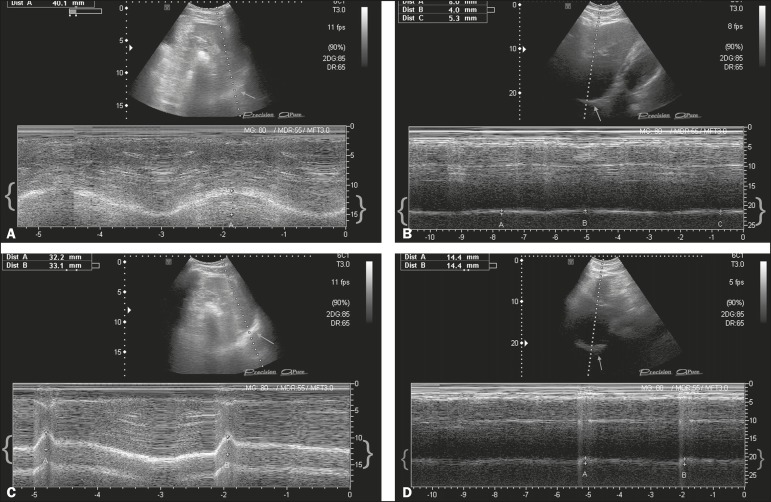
A: M-mode ultrasound during quiet breathing. Left hemidiaphragm.
